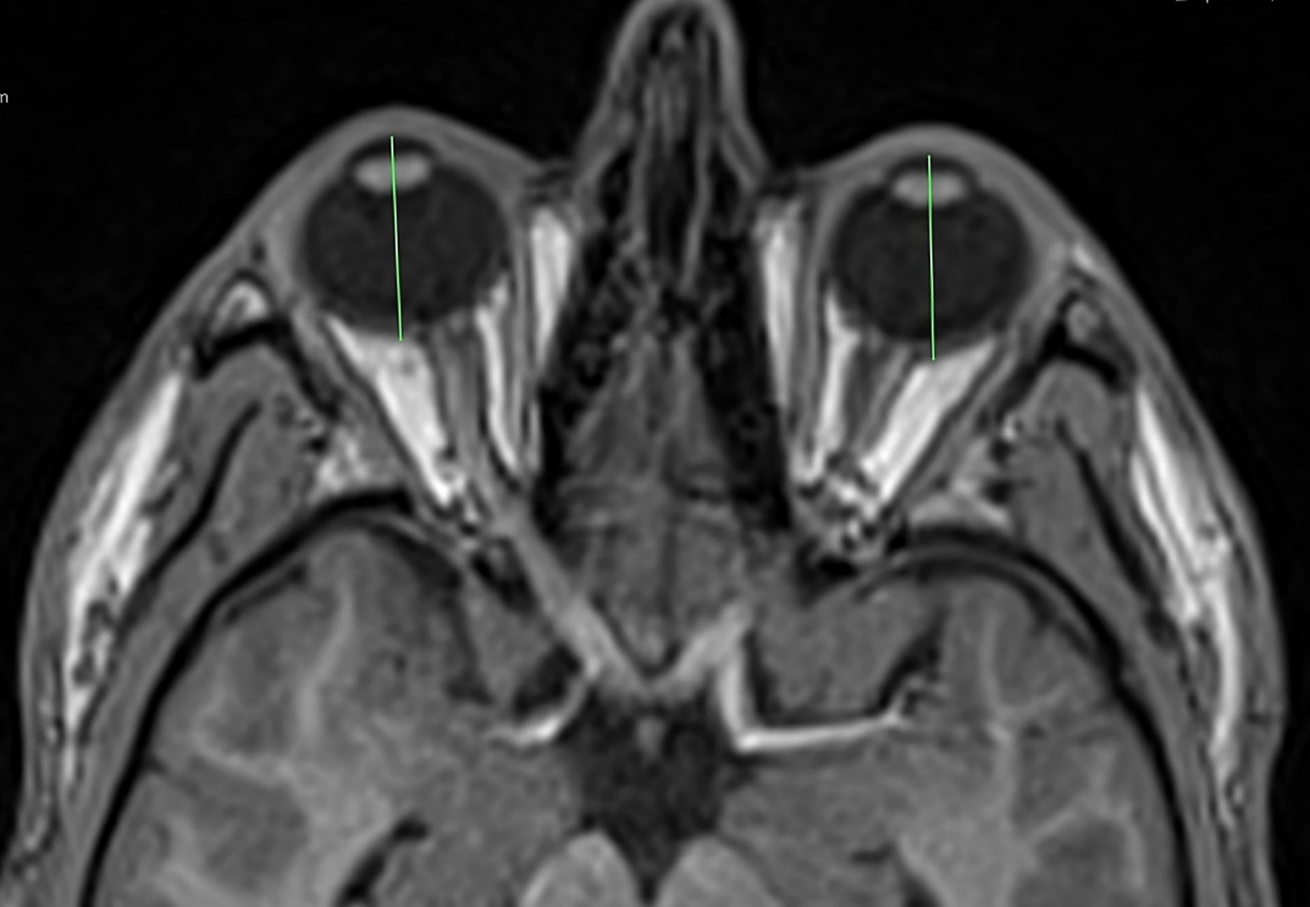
MRI-measurement of axial length.
